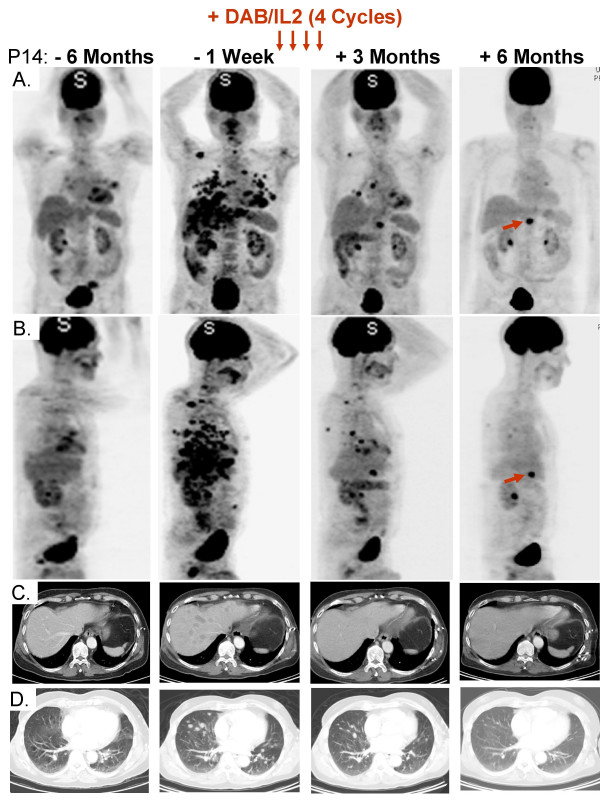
Near complete response of widespread visceralmelanoma metastases after 4 cycles of DAB/IL2. A. Anterior/posterior views, PET. B. Lateral views, PET. C. CT imaging, liver. D. CT imaging, lungs. Combined PET/CT imaging of patient P14 revealed rapid progression of multiple melanoma metastases in the liver, both lungs, lymph nodes and the subcutaneous compartment (compare -6 months to -1 week). After 4 cycles of DAB/IL2, the liver metastases completely resolved and the lung metastases markedly regressed (compare -1 week to +3 months). Three months after completion of DAB/IL2, the residual lung metastases had completely resolved but a single enlarged peri-aortic lymph node persisted (red arrow). The increased ^18^F-fluorodeoxyglucose uptake in the brain, bladder and both kidneys are due to normal metabolism and are not reflective of metastases.
